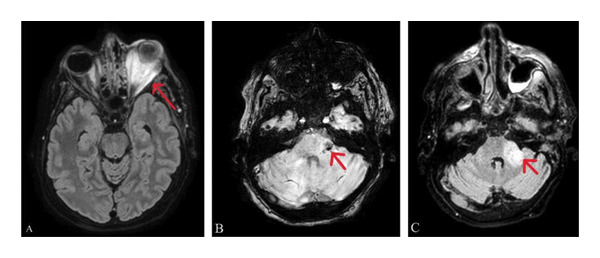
(A) Axial FLAIR image showing severe left orbital inflammation with panophthalmitis. (B) Axial SWI and (C) FLAIR images showing pontine and left middle cerebellar peduncle involvement with hemorrhagic foci.
